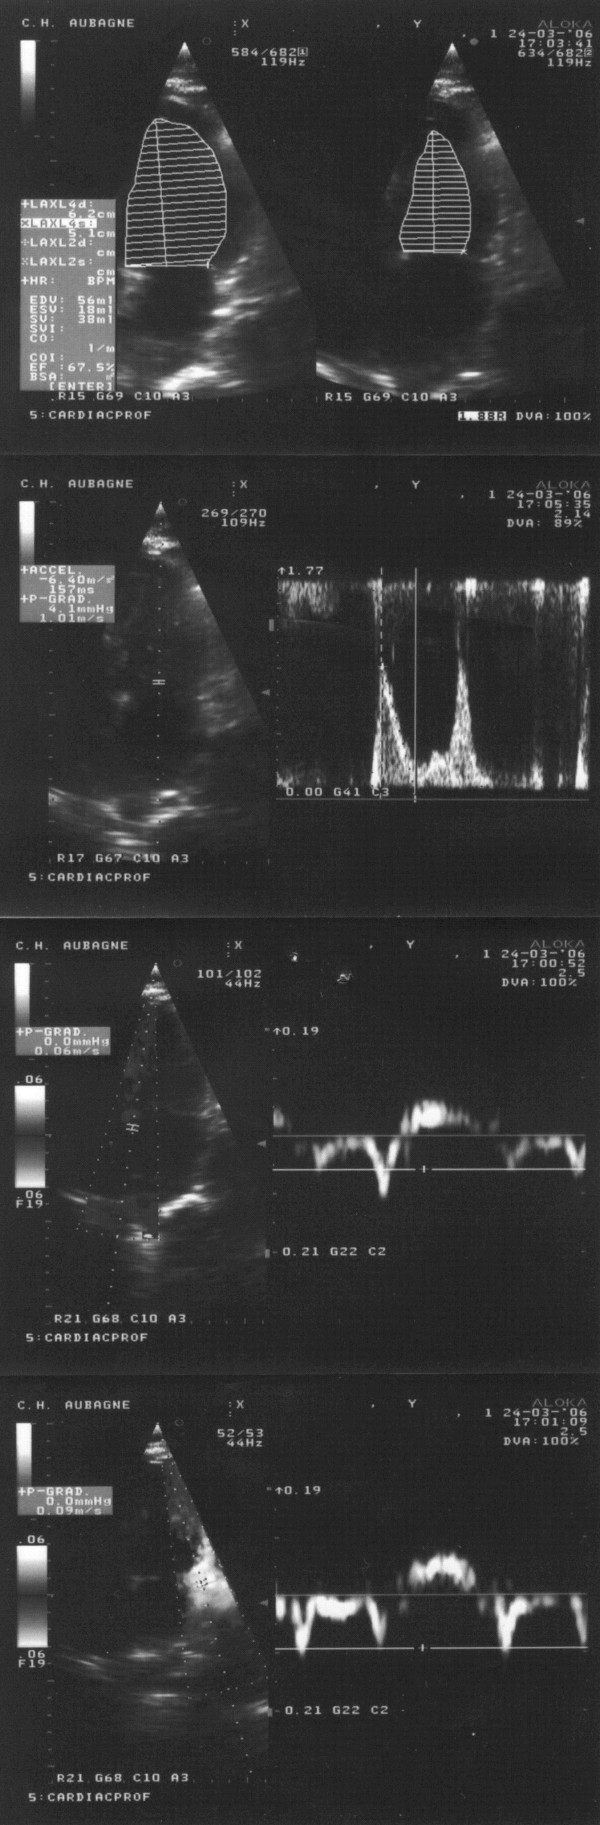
Example of bedside measurement of spectral Doppler velocities (cm/s) in the apical 4-chamber view in a 82 years-old woman hospitalized for new-onset congestive heart failure with preserved left ventricular systolic function related to longstanding hypertension. At presentation, the score of Boston criteria was 10 and B-type natriuretic peptide concentration 460 pg/ml. Bedside Doppler echocardiography performed before unloading therapy showed a left ventricular ejection fraction of 67% (upper part); peak E mitral velocity between the tips of mitral leaflets was 101 cm/s, spectral tissue Doppler peak early diastolic E' velocities at the septal (middle part) and lateral corner of mitral annulus (lower part) were 6 and 9 cm/s, respectively. The patient experienced a complete relief of symptoms and signs of pulmonary congestion under unloading therapy. Invasive left ventricular end-diastolic pressure recorded after clinical stabilization was 14 mm Hg.
